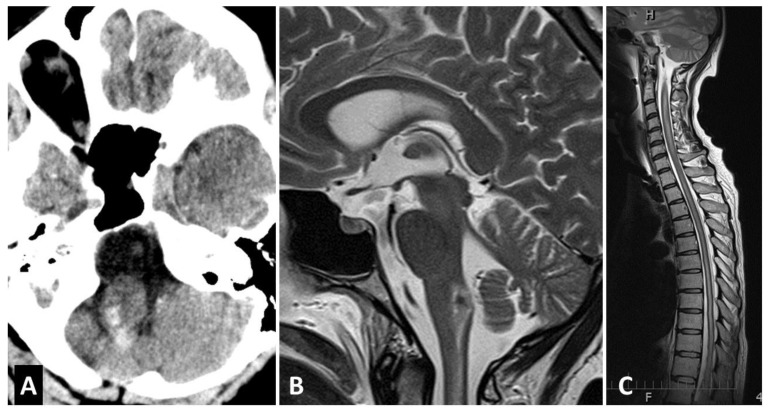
Brainstem hemorrhage. (A) Axial CT showed brainstem hemorrhage, (B,C) Sagittal T2—weighted MRI confirmed brainstem hemorrhage and revealed cervical and thoracic myelopathy.
